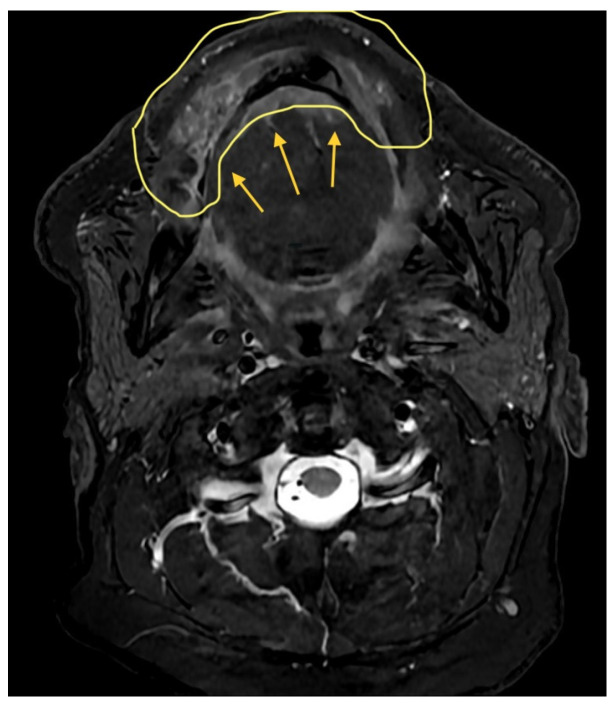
Case 1—Preoperative MRI. Yellow arrows and circle indicate the primary tumor extension.
